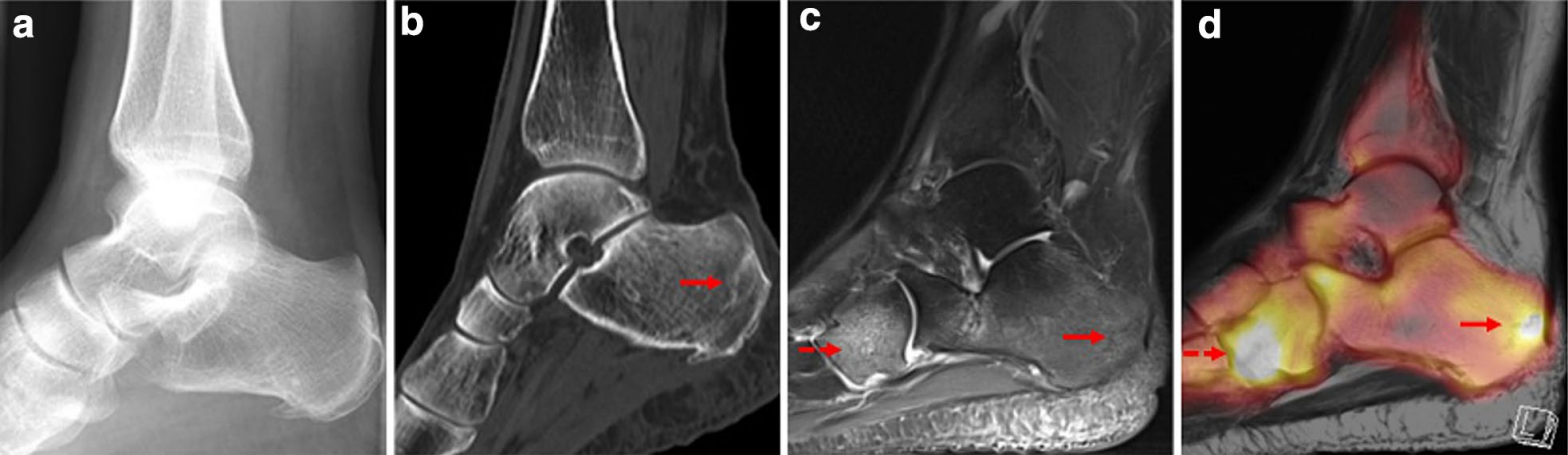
X-ray (a), CT scan (b), MRI scan (c) and ^18^F-Fluoride PET-MR (d) images of a 58-year-old female patient (patient no. 13). In the lateral X-ray (a) of the ankle region, neither acute stress fractures, nor degenerative lesions can be diagnosed. Sagittal CT images (b) show sclerotic lesions in the dorsal calcaneus (*red arrow*) and degenerative changes in the talonavicular region, no acute stress fractures are shown. Sagittal MRI images (c) show a fracture line in the dorsal calcaneus with little oedema (*red arrow*), due to an older stress fracture, coming along with a higher fluoride uptake in the ^18^F-Fluoride PET-MR (d, *red arrow*). Another stress fracture is shown in the mediodorsal parts of the cuboid with bone marrow oedema in the MRI scan (c, *dotted arrow*) and a higher fluoride uptake in the ^18^F-Fluoride PET-MR (d, *dotted arrow*)
